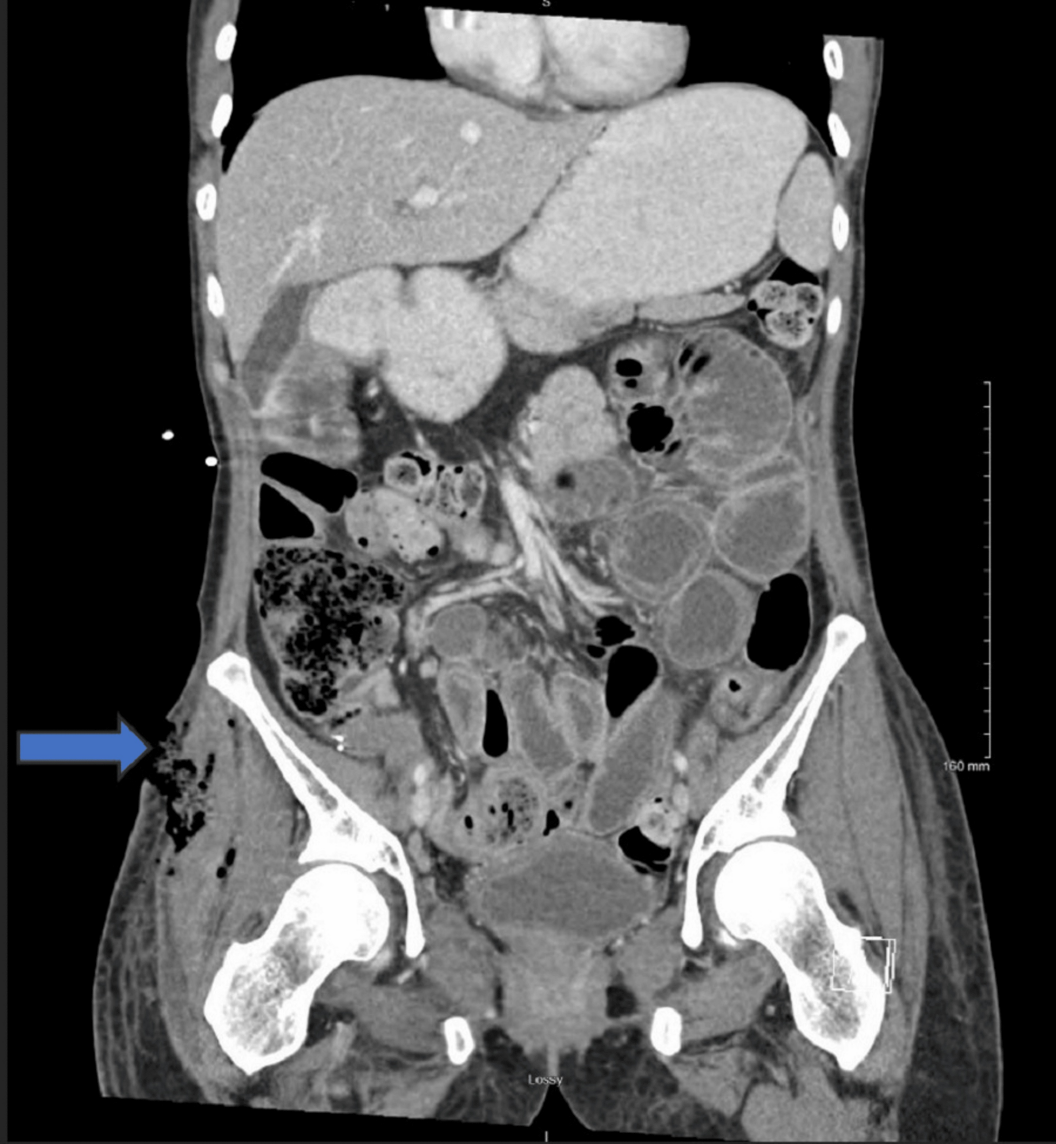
Coronal CT image of the pelvis demonstrating progression of the pelvic fluid collection with clear evidence of a fistulous connection between the bowel and the surgical wound. The blue arrows indicate the fistula tract CT: computed tomography
